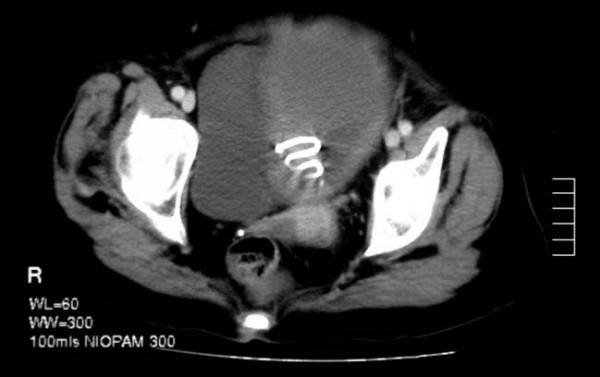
CT scan showing large pyometra with intra-uterine contraceptive device in-situ.
